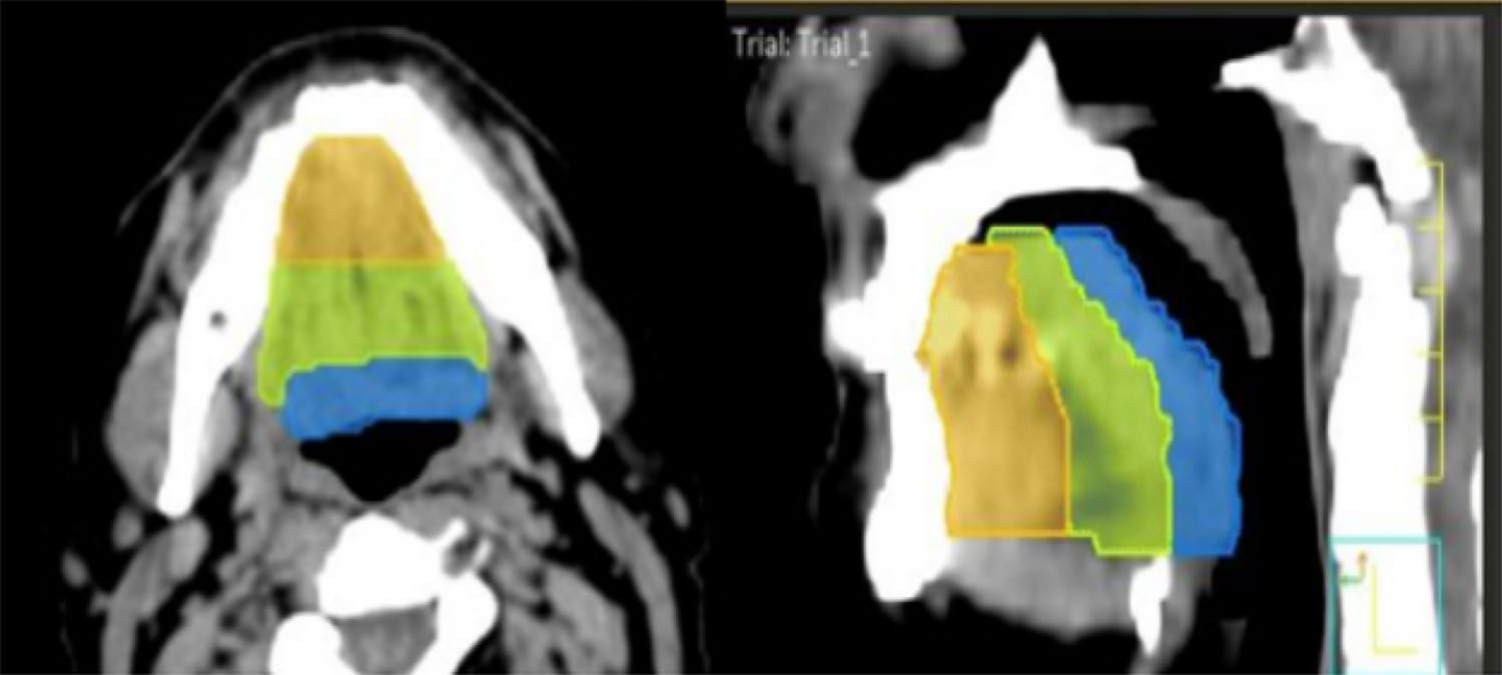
Contouring of the base (blue), medium third (green) and anterior third (yellow) of the tongue in axial (left) and sagittal (right) view on planning CT scan
